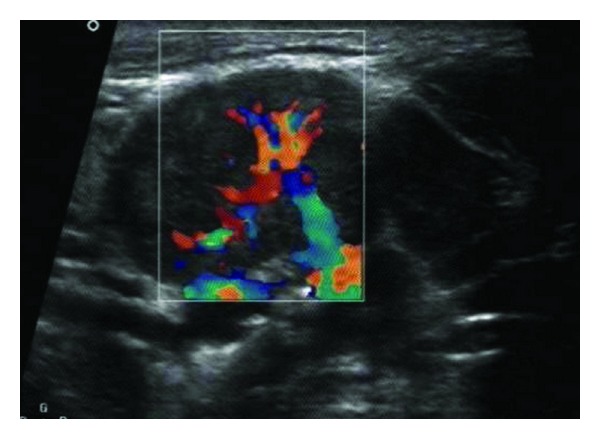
Lesion within left parotid on colour doppler study; shows significant vascularity.
